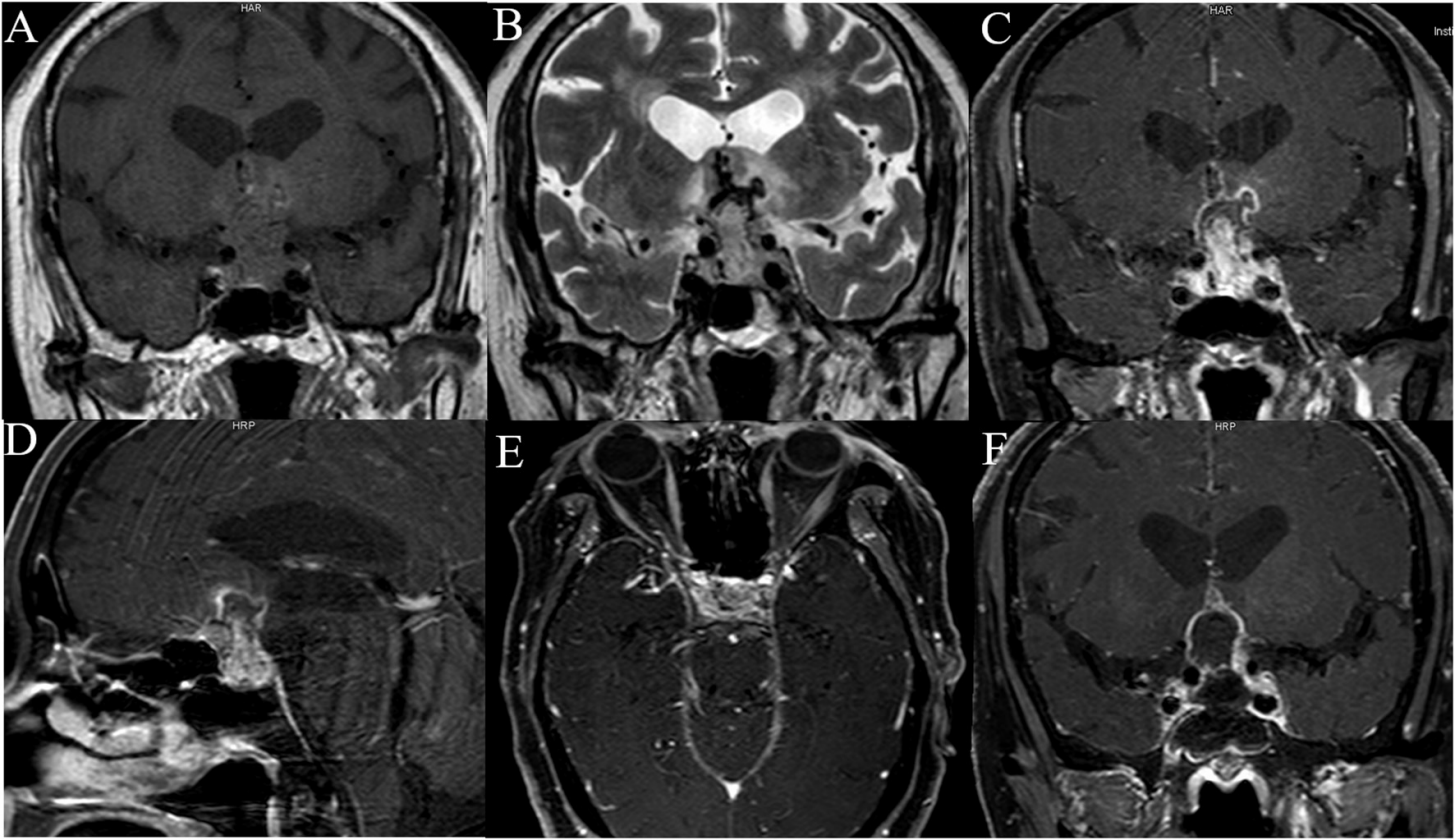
The neuroimaging of case 5. Pre-operative T1-weighted image (A), T2-weighted image (B), coronal (C), sagittal (D), axial (E), T1-weighted contrast-enhanced MRI of case 5 show intrasellar heterogeneously enhanced solid and cystic mass with suprasellar extension compressing and encasing the optic chiasm, invasion of bilateral cavernous sinus. Post-operative MRI scan of the brain shows subtotal resection of the tumor (F).
